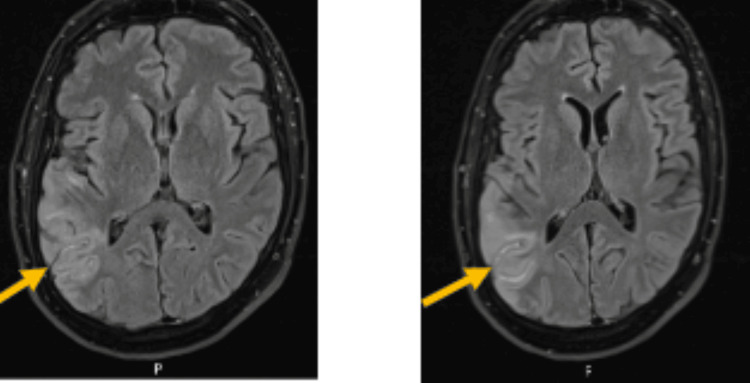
MRI head sub-acute right MCA territory infarct. Area of increased FLAIR signal involving the cortex and subcortical white matter of the posterior right parietal and right temporal lobes. MCA: middle cerebral artery; FLAIR: fluid-attenuated inversion recovery
